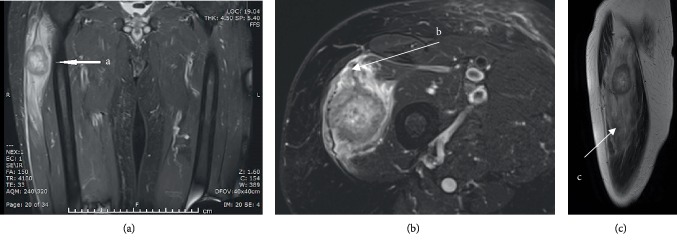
T2-weighted MRI images of the right thigh tumour. (a) Coronal image of the right thigh tumour. (b) Axial image of right thigh tumour and surrounding oedema. (c) Sagittal image of right thigh tumour with enhancing central element.
